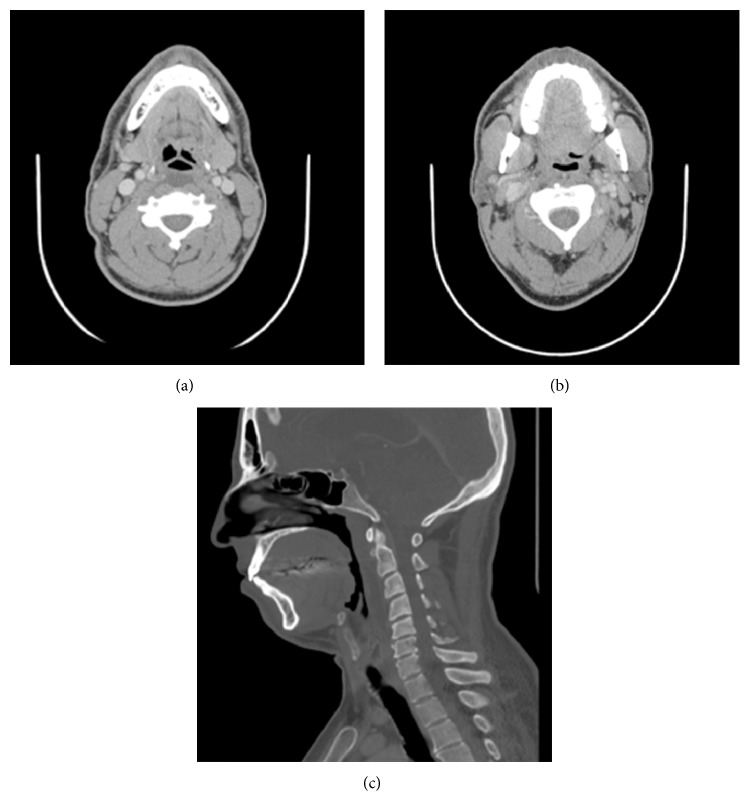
CT scan of the neck with contrast (axial and sagittal views) demonstrating a retropharyngeal fluid collection with no rim enhancement extending from C2 to C6 ((a) and (c)) and a 5 mm focus of calcification within the longus colli muscle anterior to C2 (b) shortly after initial presentation and prior to treatment.
